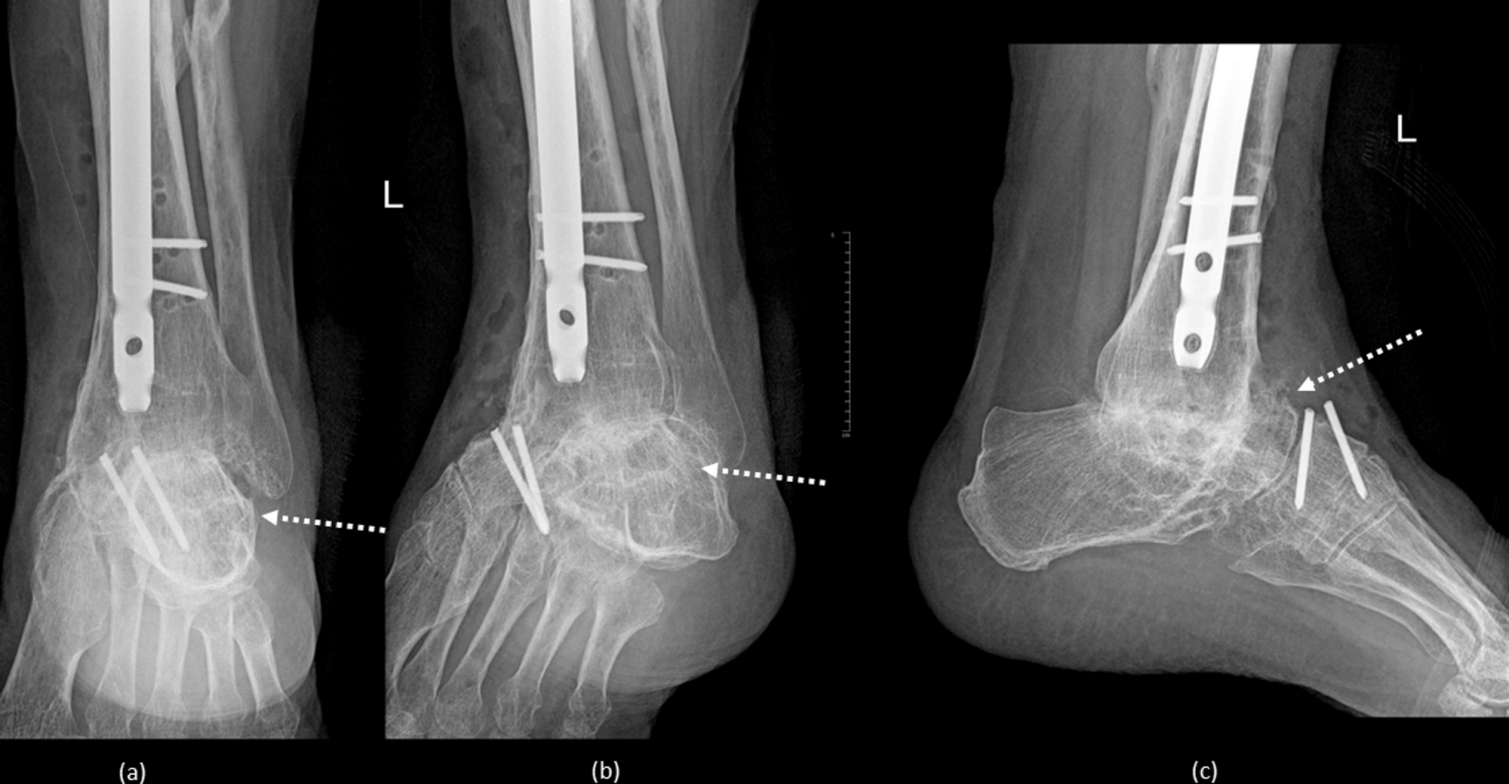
Radiographs post-removal of material and complete fusion of the tibiocalcaneal arthrodesis: (a) anteroposterior view, (b) oblique view, and (c) lateral view.
